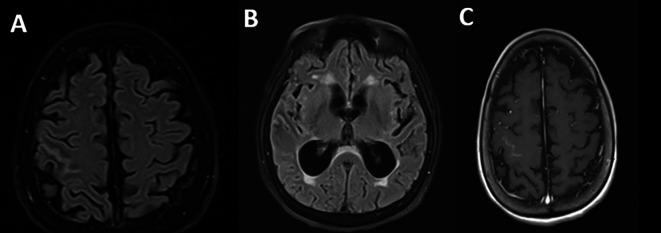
Brain magnetic resonance imaging: A. Irregular leptomeningeal thickening at the right Rolando fissure (thickness 2 mm), as well as smaller adjacent focal parietal leptomeningeal thickening (FLAIR sequence); B. Supratentorial hydrocephalus of recent onset associated with interstitial/transependymal edema (FLAIR sequence); C. No expansive lesions or enhancements in the brain parenchyma (T1 with contrast).
